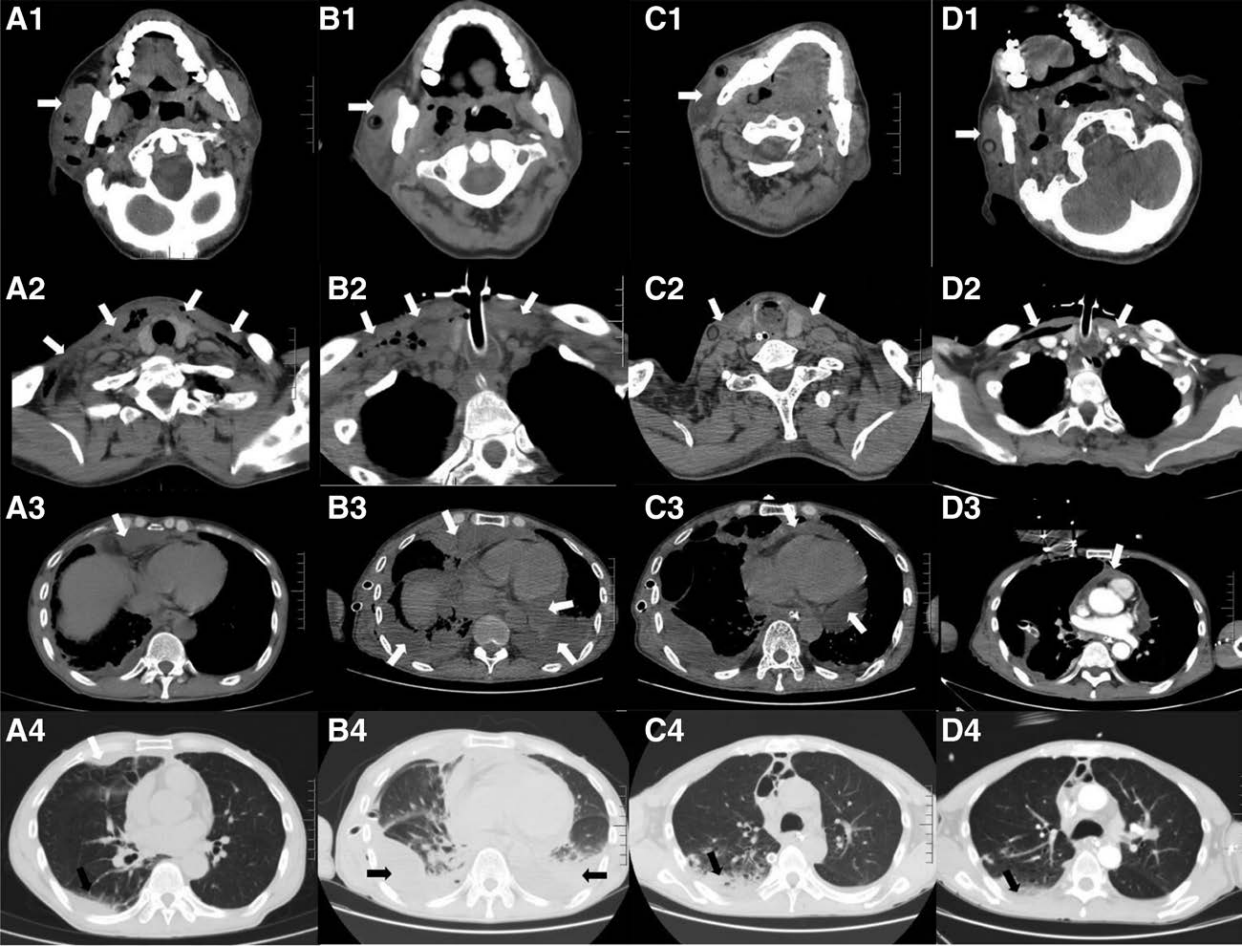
Head and chest CT images at different time-points. (A) CT images at admission; (B) CT images after surgery; (C) CT images after surgical and antibiotic treatment; (D) CT images at discharge. White arrow indicates the abscess, effusion, and pneumatization. Black arrow indicates the lung exudation. CT = computed tomography.
